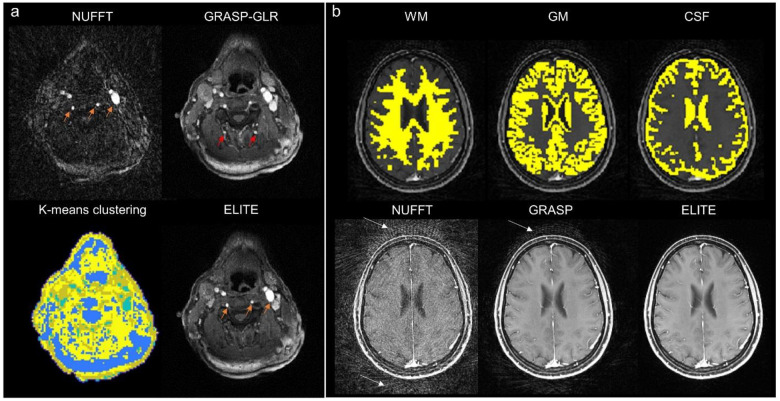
Applications in DCE-MRI of the head and neck and the brain. Other anatomical information can be utilized for ELITE image reconstruction: (a) ELITE DCE head and neck data yields time-series images with accurate temporal enhancement of arterial and venous blood, consistent with NUFFT (orange arrows), while GRASP-GLR exhibits a false enhancement in posterior vessels (red arrows). (b) Brain imaging can also be utilized for ELITE segmented-based reconstructed composed of white matter (WM), gray matter (GM) and cerebrospinal fluid (CSF) maps, resulting in enhanced T1-weighted anatomical images with reduced streak artifacts (white arrows).
